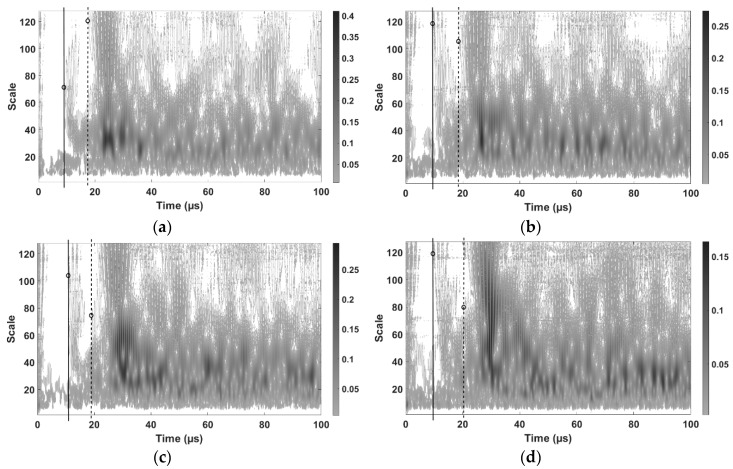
Time-frequency maps of ultrasonic waves induced at different scan points. The dotted line, represents direct ultrasonic wave arrival, the solid line, ultrasonic defect-reflected echo arrival. The corresponding ultrasonic transducer position is *x* = 15 mm and *y* = 110 mm, and the positions of laser scan points are: (a) *x* = 11 mm and *y* = 71 mm (b) *x* = 11 mm and *y* = 51 mm. (c) *x* = 31 mm and *y* = 41 mm. (d) *x* = 81 mm and *y* = 41 mm.
